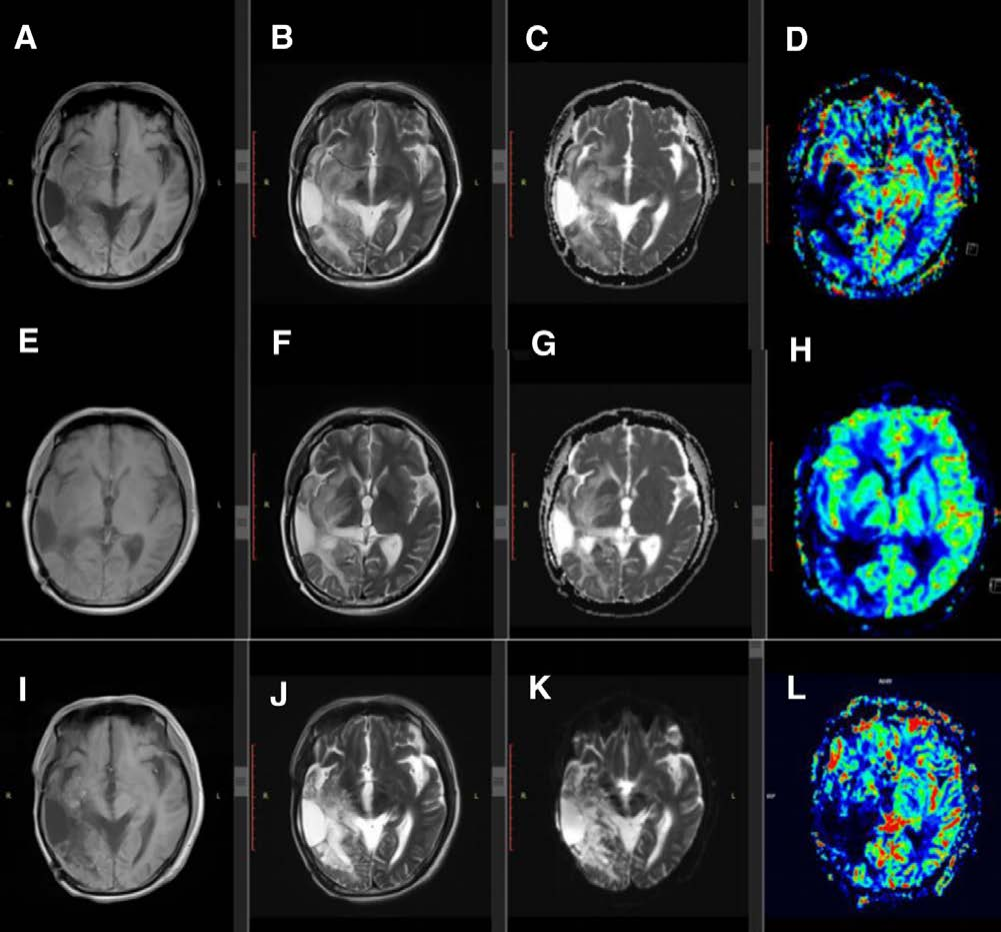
Magnetic resonance imaging on July 10, 2017. (A: T1W; B: T2W; C: ADC; D: PWI). On January 02, 2018 MRI (E: T1W; F: T2W; G: ADC; H: PWI). On May 12, 2020, MRI (I: T1W; J: T2W; K: ADC; L: PWI). PWI = perfusion weighted imaging.
